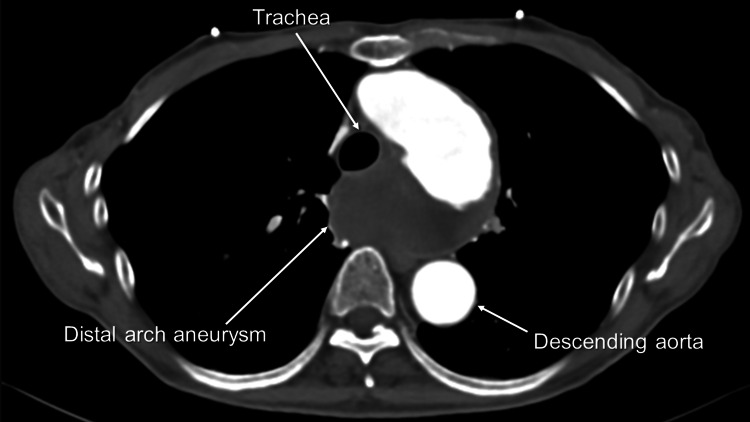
Preoperative contrast-enhanced computed tomography (CT) revealing a distal arch aneurysm
